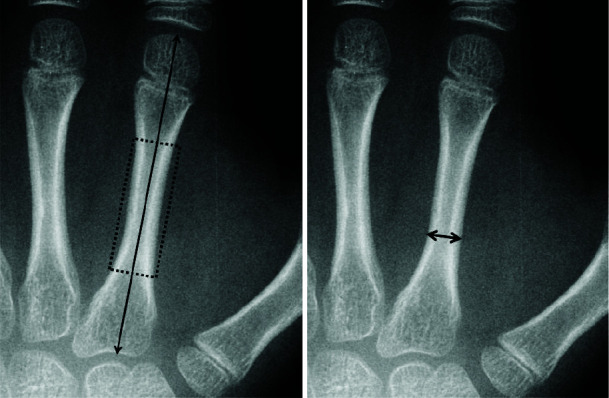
2^nd^ metacarpal bones length and width in hand radiographs
